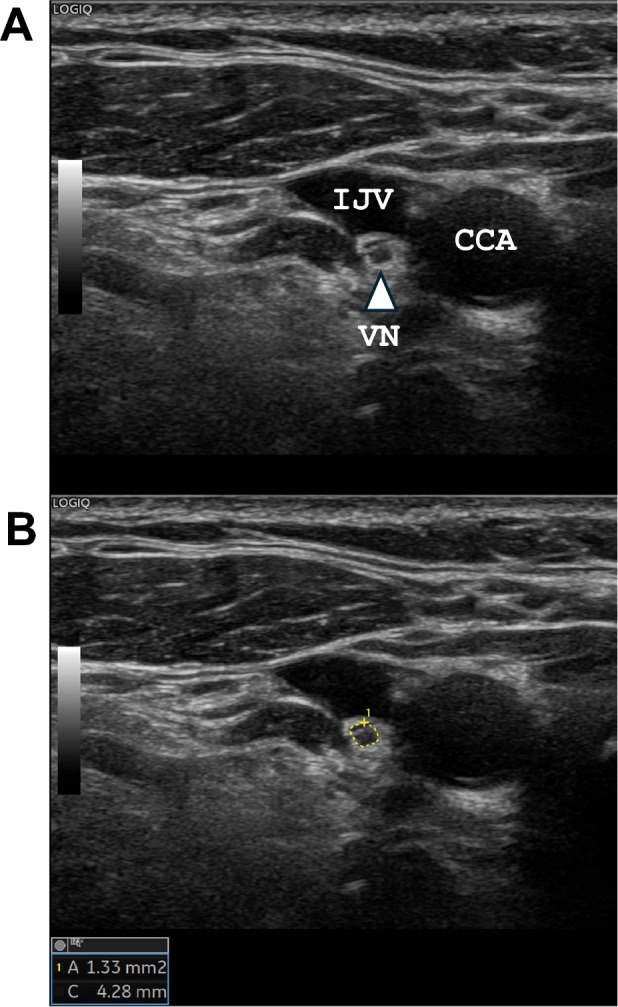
Ultrasonographic image of the vagus nerve (VN). The right VN is shown as a small, rounded, hypoechoic structure between the common carotid artery (CCA) and the internal jugular vein (IJV) (A, triangle arrow). The cross-sectional area of the VN was measured by manual tracing (B, yellow circle)
